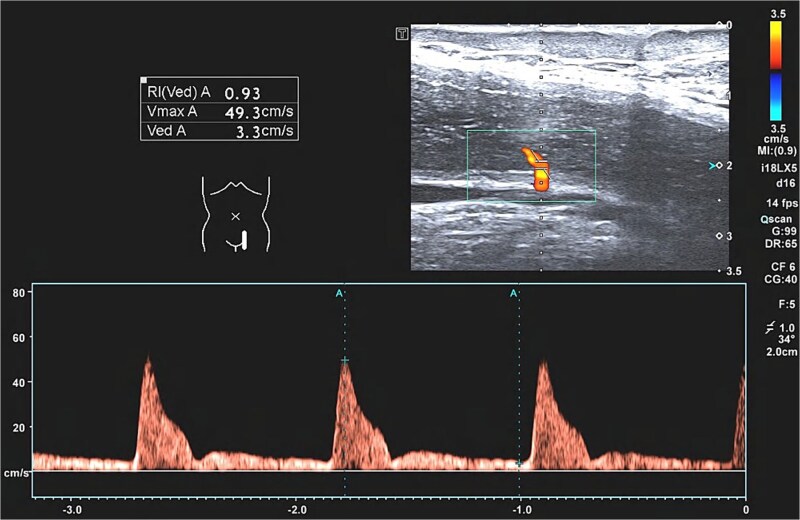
Color doppler ultrasound examination and measurement of CCP root penile artery.
